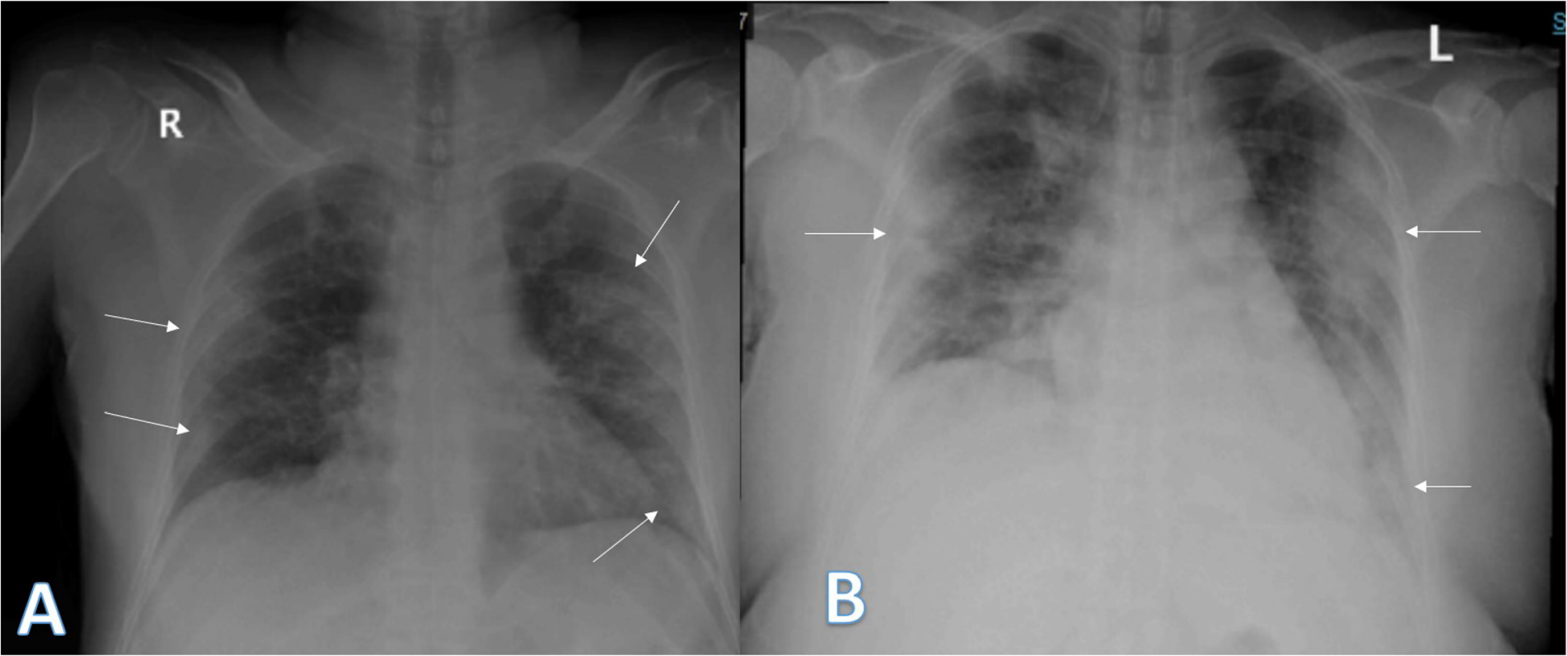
Two different patients with positive COVID-19. a 52-year male was complaining of fever, SOB, and cough. Initial chest X-ray showed right (peripheral) and left mid and lower zonal air space consolidation opacities with midzonal predominance (arrows). The severity score was 2 for the right lung and 3 on the left lung, so TSS was 5. b 36-year female was complaining of cough and fever. Initial chest X-ray showed mid and lower zonal air space consolidation opacities on both lungs with midzonal predominance (arrows). The severity score was 2 for the right lung and 3 on the left lung, so TSS was 5
